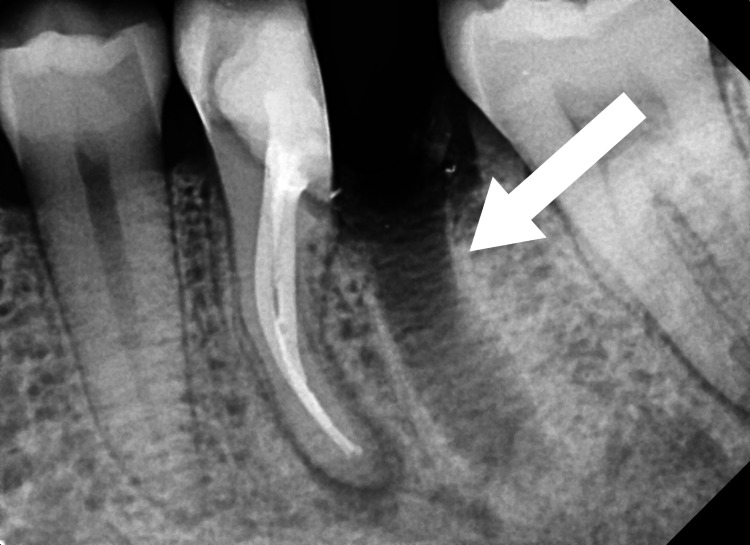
Immediate post-operative radiograph showing the clear extraction socket following the removal of the distal segment of tooth number 36
